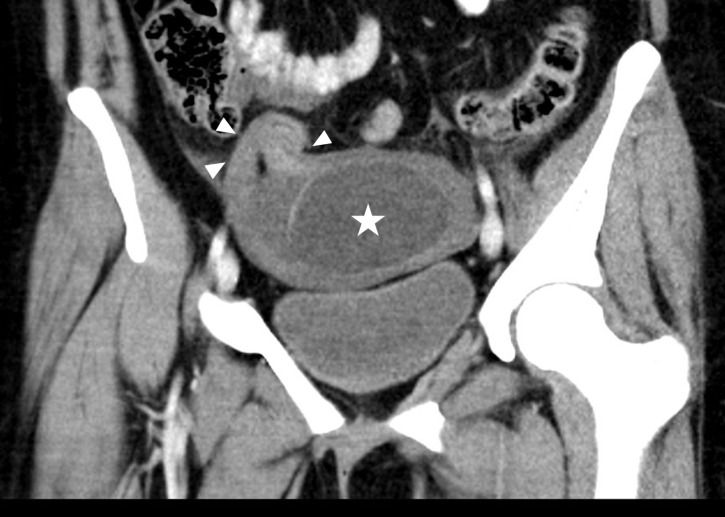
Coronal computed tomography of the pelvis shows the 7-centimeter cystic structure (star) within the pelvis with surrounding fluid. Along the right side of the cystic structure there is extension into the location of the area of the right fallopian tube (arrowheads).
